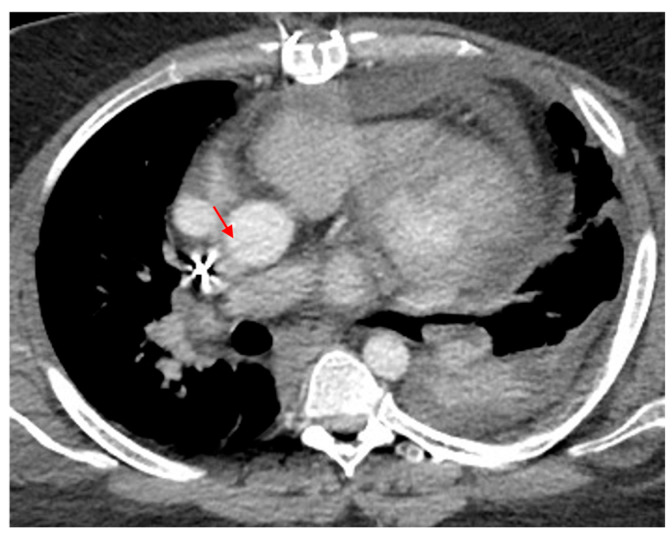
Metal artifact. Axial cardiac CT image shows a metallic artifact (red arrow) from a central venous catheter.
